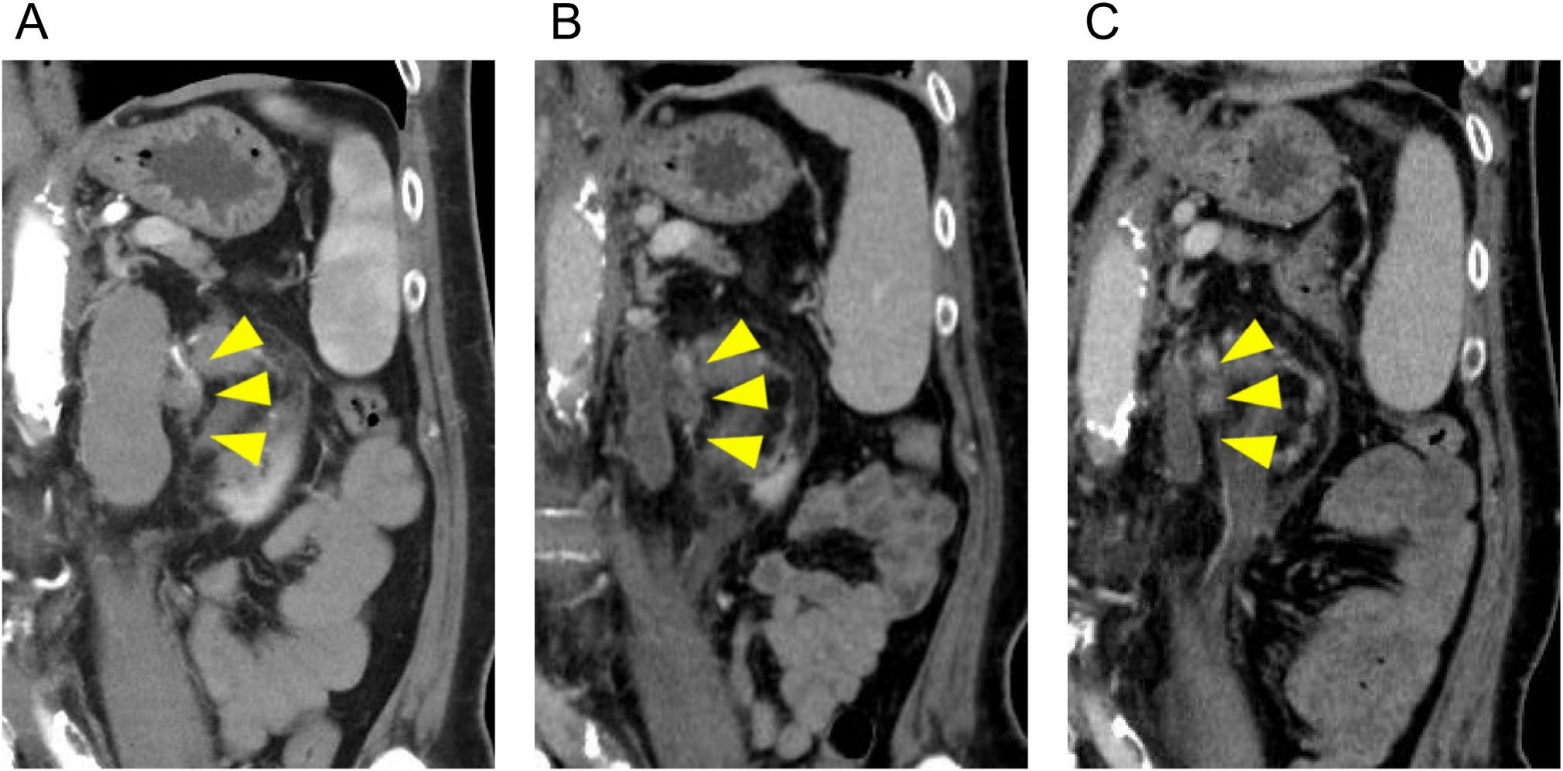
Dynamic computed tomography images of renal hilar lymph node: (A) before treatment with pembrolizumab plus lenvatinib, (B) 2 months after initiation of the combination therapy, and (C) 6 months after initiation of the combination therapy.
